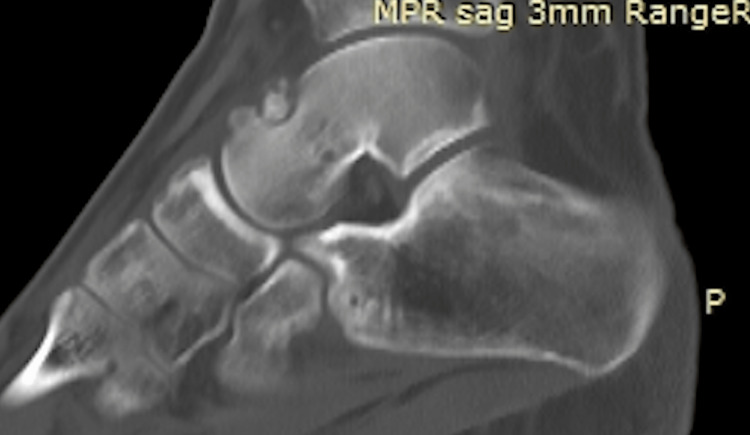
An image from a CT scan demonstrating an osteoid osteoma of the talar neck.
